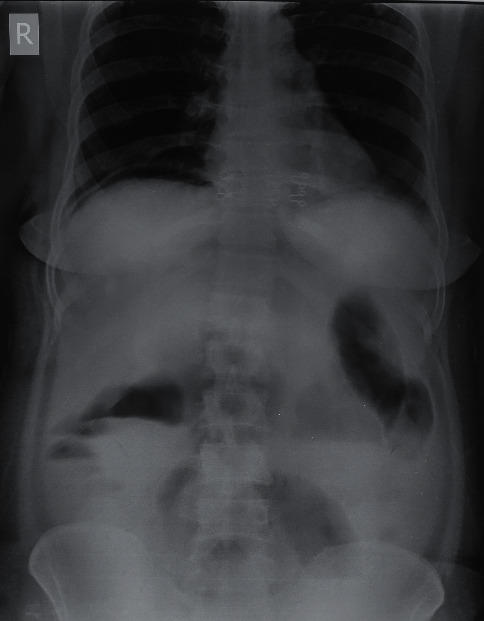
X-ray abdomen showing free gas under right dome of diaphragm.
